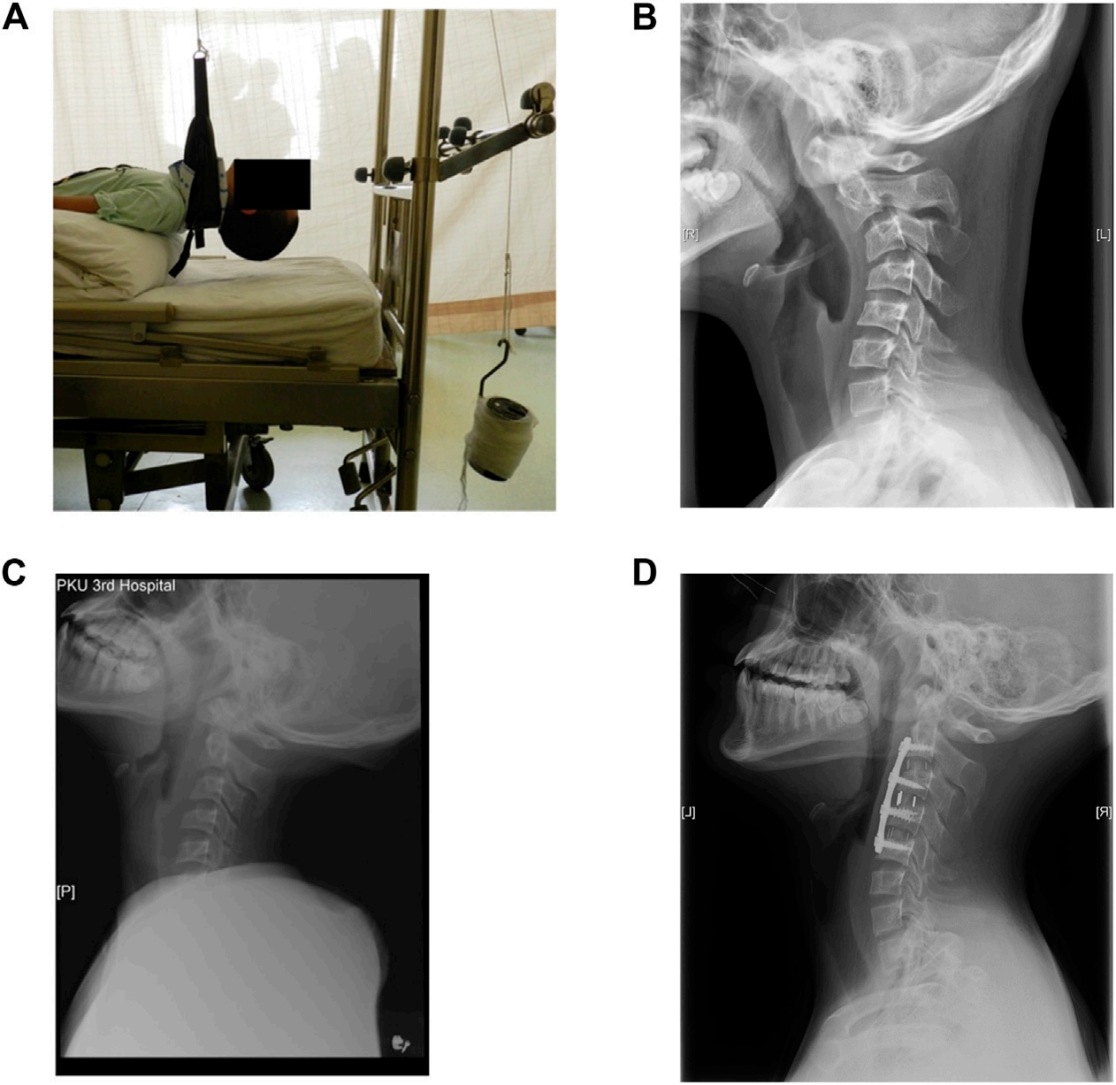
(A) Photo of the preoperative moment-balanced traction, (B) lateral view of preoperative cervical X-ray, C2–C5 Cobb angle was −53.2°, (C) X-ray after the moment-balanced traction, (D) lateral view of cervical spine at three-month follow-up, Cobb angle of C2–C5 was −3.3°.
